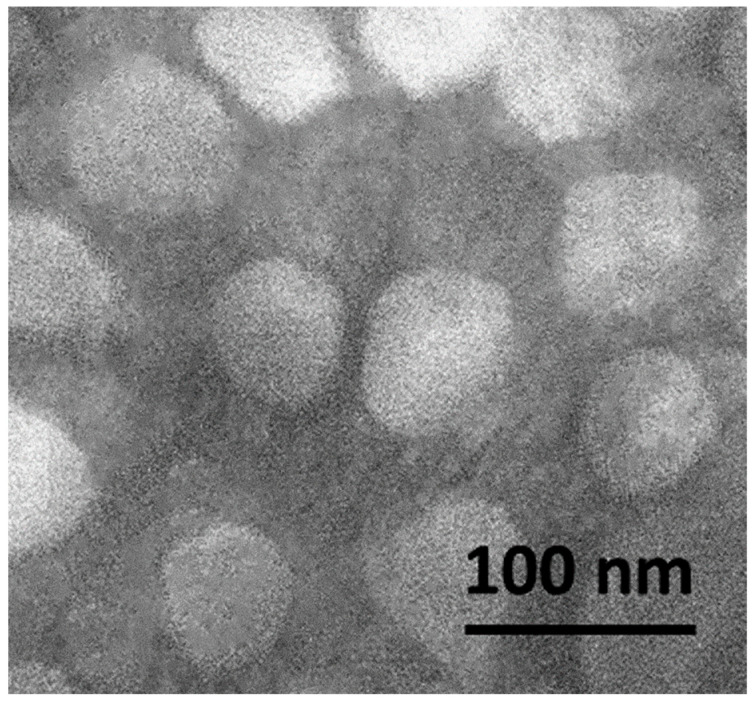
SEM image of HA–CT–B12 conjugate.
